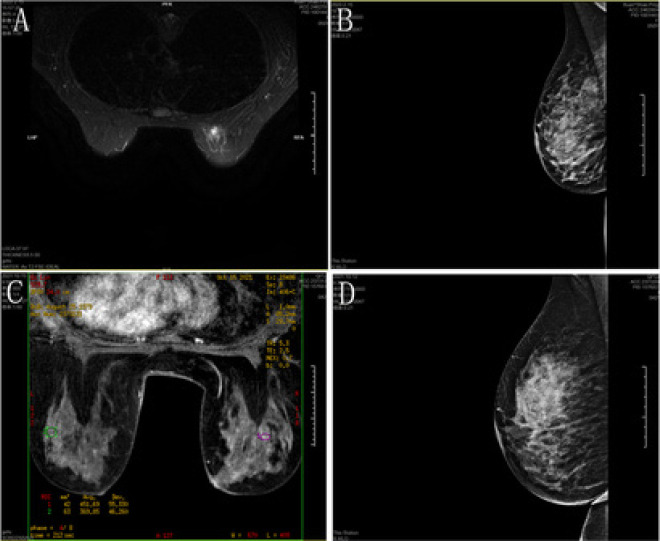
Typical case figures. A: DCE-MRI for breast cancer: Multiple dot-, sheet- and cord-like T2FS hyperechoic shadows can be observed, and nodules occupied the upper inner quadrant of the right breast, which had a size of about 18×14 mm, strong T2WI signal and weak T1WI signal. Post-enhanced scan showed obvious enhancement in the early stage. B: Mammography for breast cancer. C: DCE-MRI for benign breast lesion: Mammary glands on both sides were ACR3-type. There was strong patch- and cord-like T2fs signals in the areola and upper outer quadrant, and the signal with DWIb value of 500-800 was weakened. There was an irregular mass in the outer upper part of the right breast close to the axillary area, and the diffusion signal was enhanced with increasing b value. The enhanced scan showed rapid inflow and outflow enhancement, and the patch-like nodules in the mammary glands on both sides all exhibited inflow enhancement, without enlarged lymph nodes in both armpits. D: Mammography for benign breast lesion
